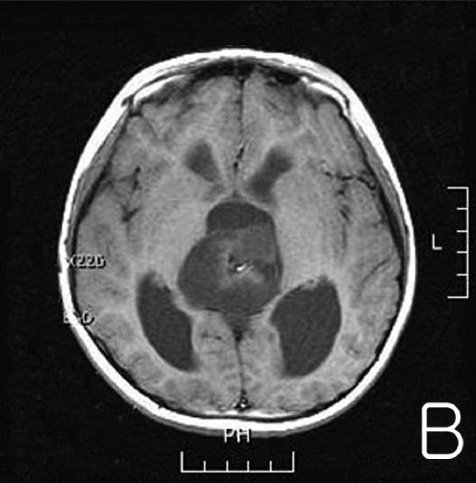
T1-weighted gadolinium-enhanced axial magnetic resonance (MR) images (B) showing an inhomogenous enhancing mass in the pineal gland
